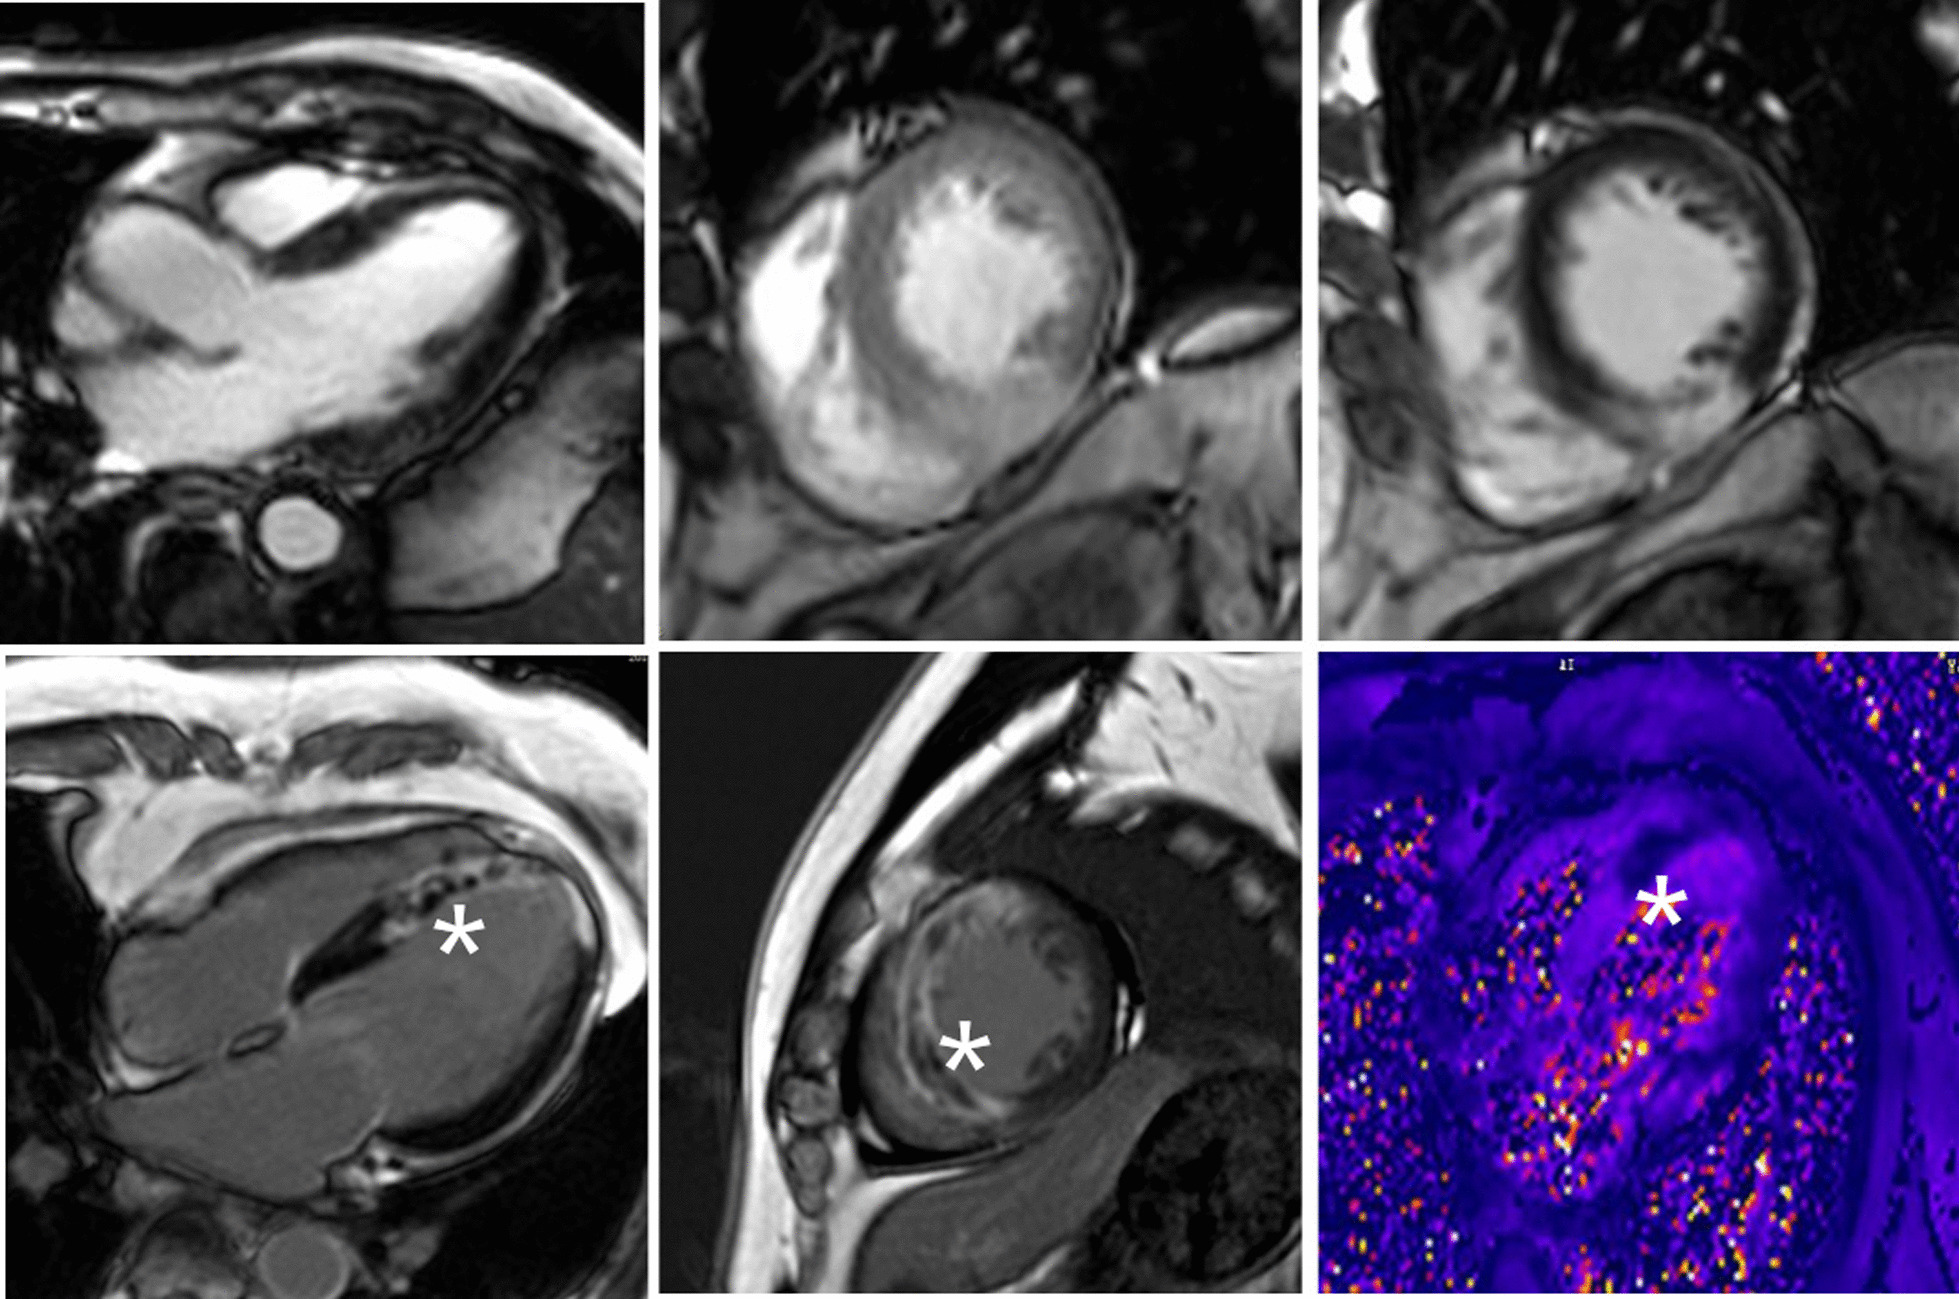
Cardiac magnetic resonance image. The top row was from a patient who under prolonged balloon inflation during stent deployment strategy, there is no imaging features of microvascular obstruction (MVO). Bottom row was from a patient with anteroseptal infarct who under conventional deployment strategy, there is imaging features of MVO (asterisk *)
